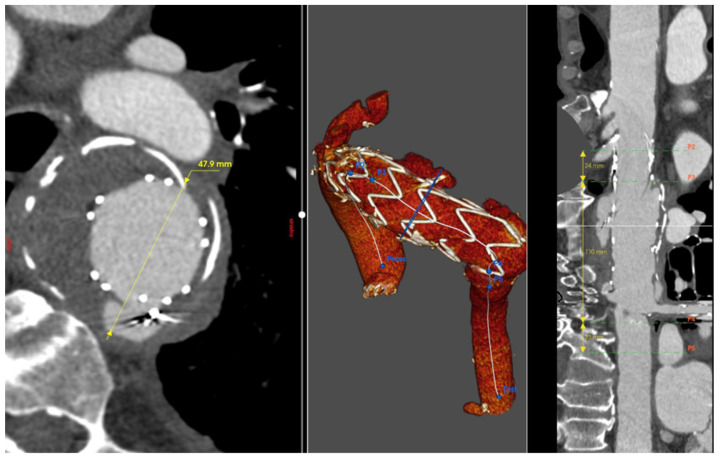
Patient 5’s Computed Tomography Angiography reconstruction showing the type III endoleak caused by rupture of the TEVAR secondary to infection.
